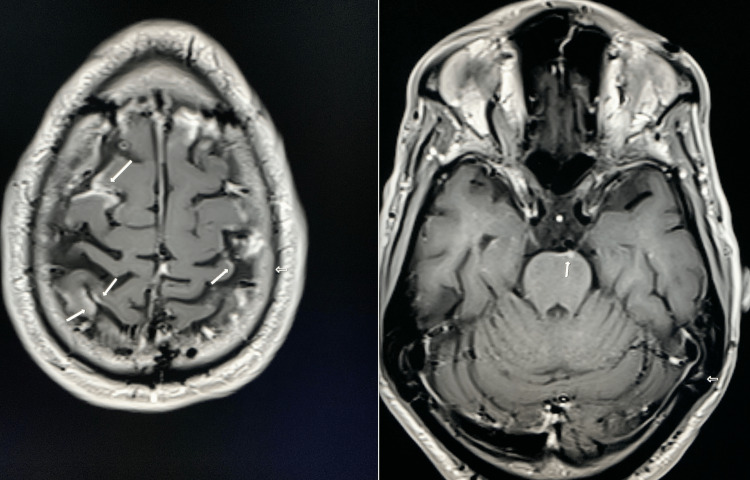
MRI Brain MRI brain demonstrating ependymal enhancement involving bilateral occipital horns (left) and leptomeningeal enhancement involving bilateral frontoparietal sulci at the vertex and ventral surface of the pons (right), representing meningitis with ventriculitis
